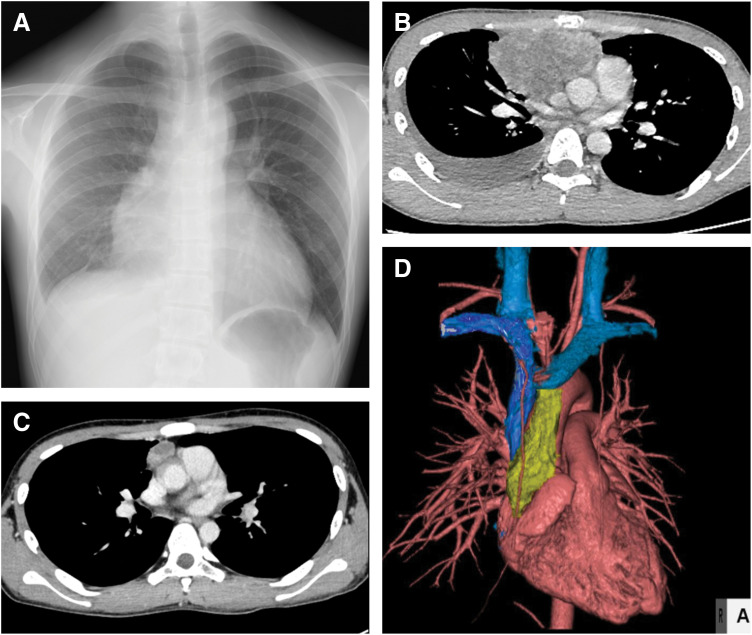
(A) Chest X-ray was taken on admission showed widening of the lower mediastinum. (B) Chest CT before chemotherapy showing a large mass in the anterior mediastinum and right pleural effusion. (C) Chest CT after chemotherapy showing that the mass had shrunk. (D) 3D reconstruction image before surgery.
